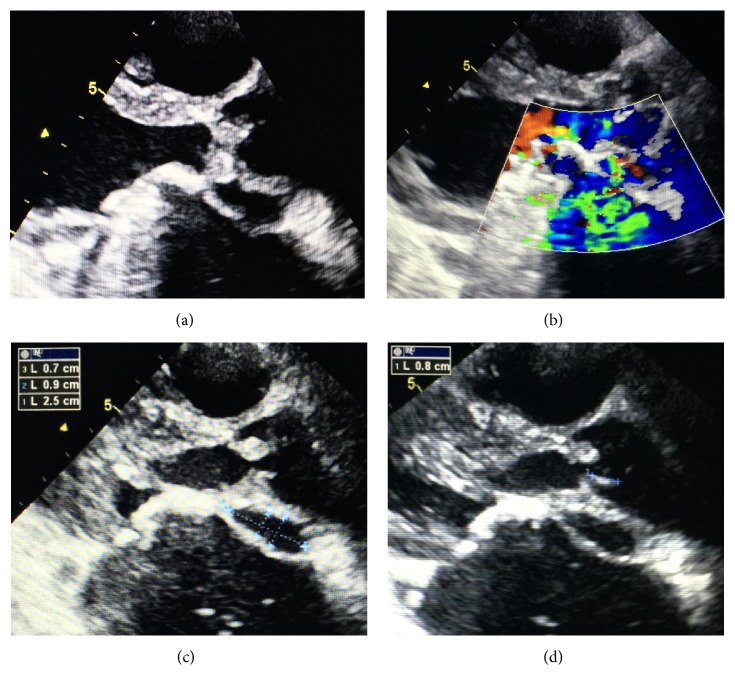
TTE: parasternal long axis view showing aortic periannular abscess communicating with the left atrium without (a, c) and with color Doppler imaging (b) and aortic vegetation (d).
